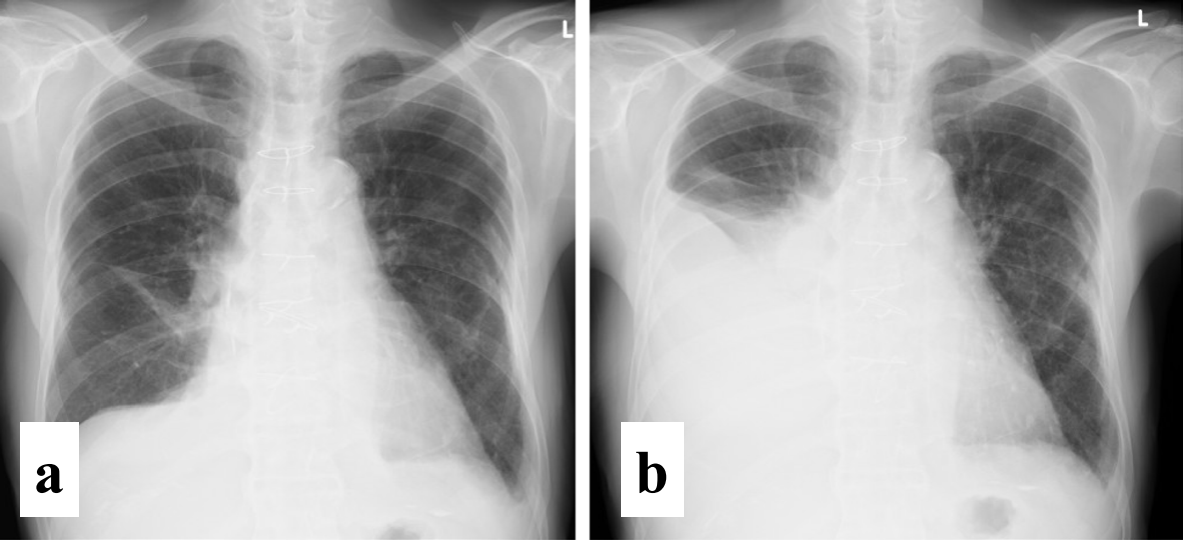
Chest radiograph. a There is no pleural effusion at the time of discharge (postoperative day 36). b A large amount of effusion is observed in the right pleural cavity at the time of readmission (postoperative day 83)
